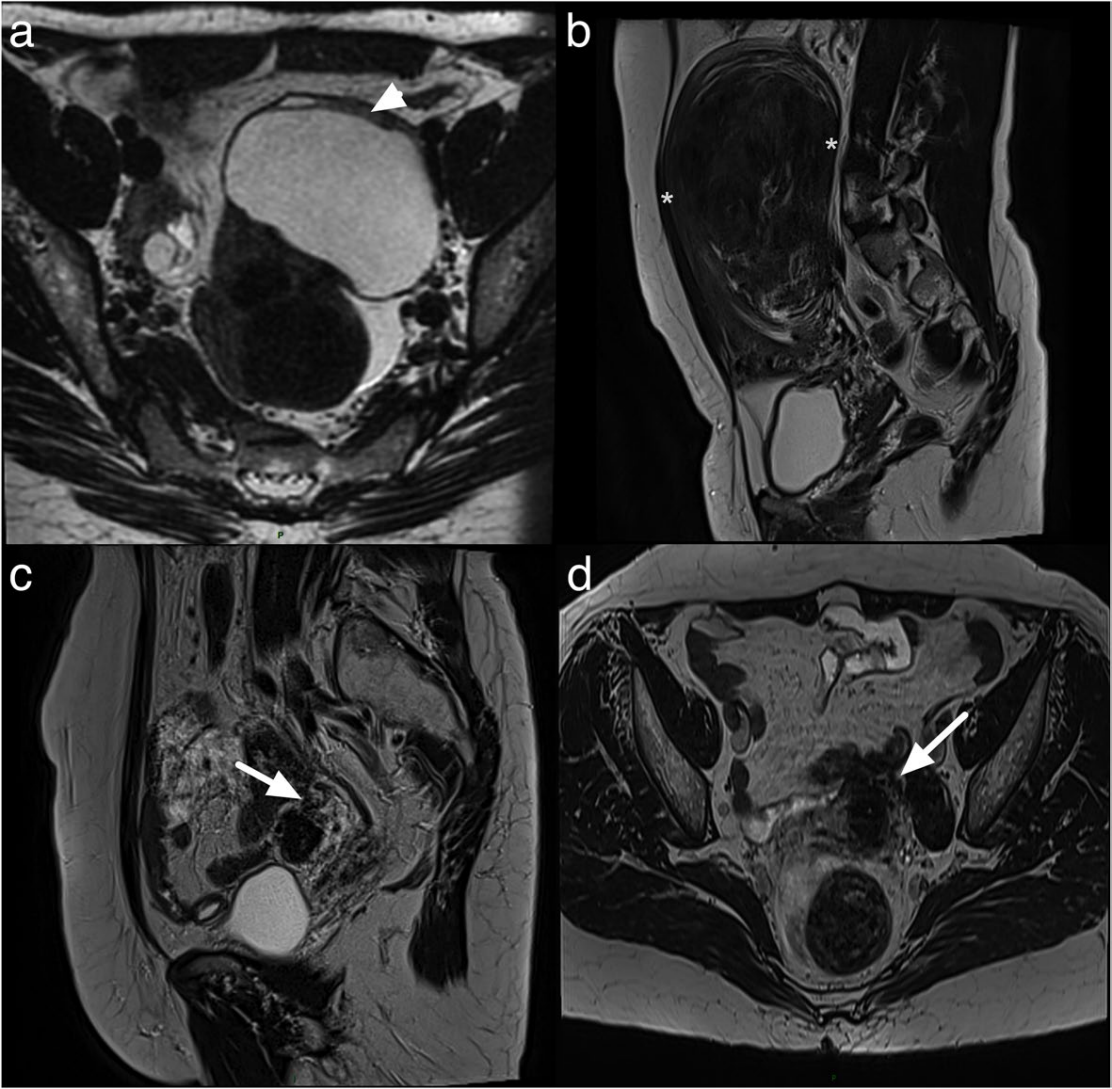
Useful sign to evaluate the relationship between a lesion and adjacent organ: a Axial T2-weighted sequence with a “beak sign” (white arrow head). b Sagittal T2-weighted sequence showing a “claw sign” (white star). c, d Sagittal and Axial T2-weighted sequence with “bridging vessel sign” (arrow)
